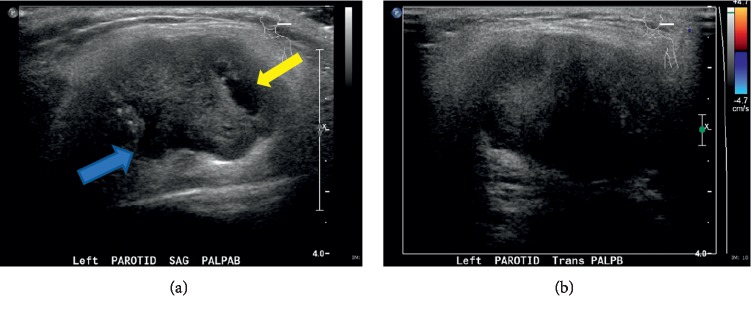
Sagittal (a) and transverse (b) ultrasound images show a well-defined lobular-shaped complex solid mass in the left parotid gland. Bright discrete echoes suggest calcifications (blue arrow; shadowing not clearly demonstrated), while an anechoic component indicates cystic fluid or necrosis (yellow arrow). Note the lack of color flow on the transverse image.
